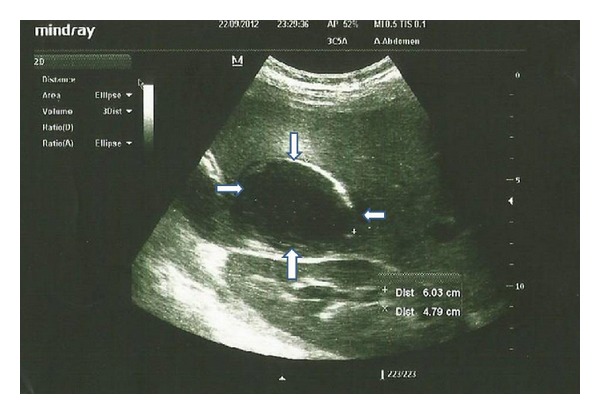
The abdominal ultrasound image of calcified wall of the abscess in 23-week pregnant woman.
